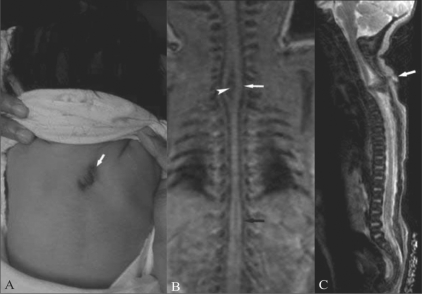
Gross examination of the neonate (A) shows swelling and a tuft of hair (white arrow) in the midline. T1W coronal MRI image (B) of the neonatal spine shows splitting of the spinal cord in the cervicodorsal (white arrow) and lower dorsal (black arrow) regions, with a thick band between the two hemicords (arrowhead). Sagittal T2W HASTE image (C) shows a track (white arrow) extending from the skin surface to the spinal canal.
